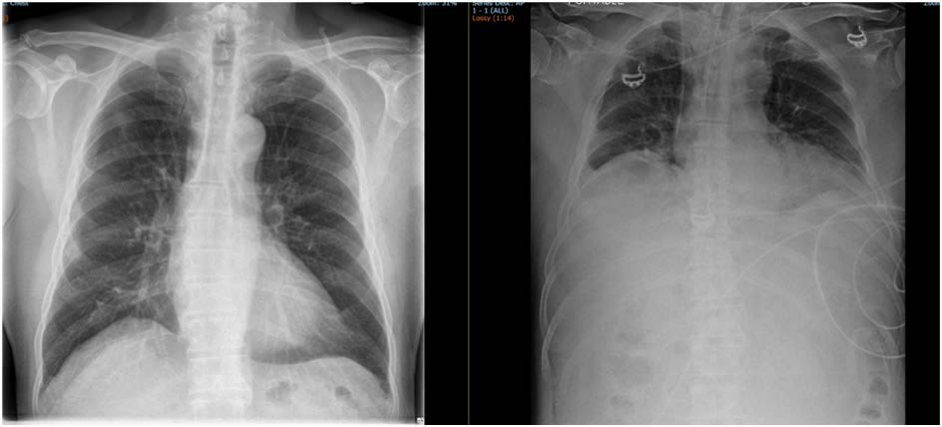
Comparison of Preoperative and Postoperative Chest X-rays in the patient with Abdominal Compartment Syndrome following HoLEP. The left image shows a normal preoperative chest X-ray, while the right image demonstrates postoperative abdominal distension and diaphragmatic splinting with reduced lung fields.
